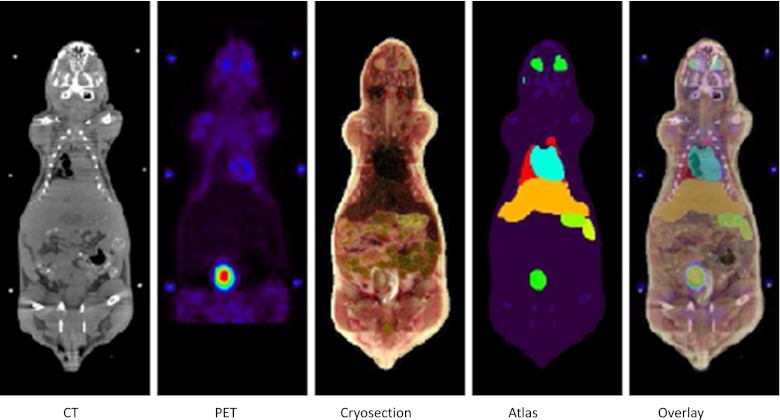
Spatially registered multimodality images for a coronal slice through the Digimouse model. From *left to right*: X-ray CT, PET, cryosection, segmented atlas and overlay of the atlas onto cryosection
